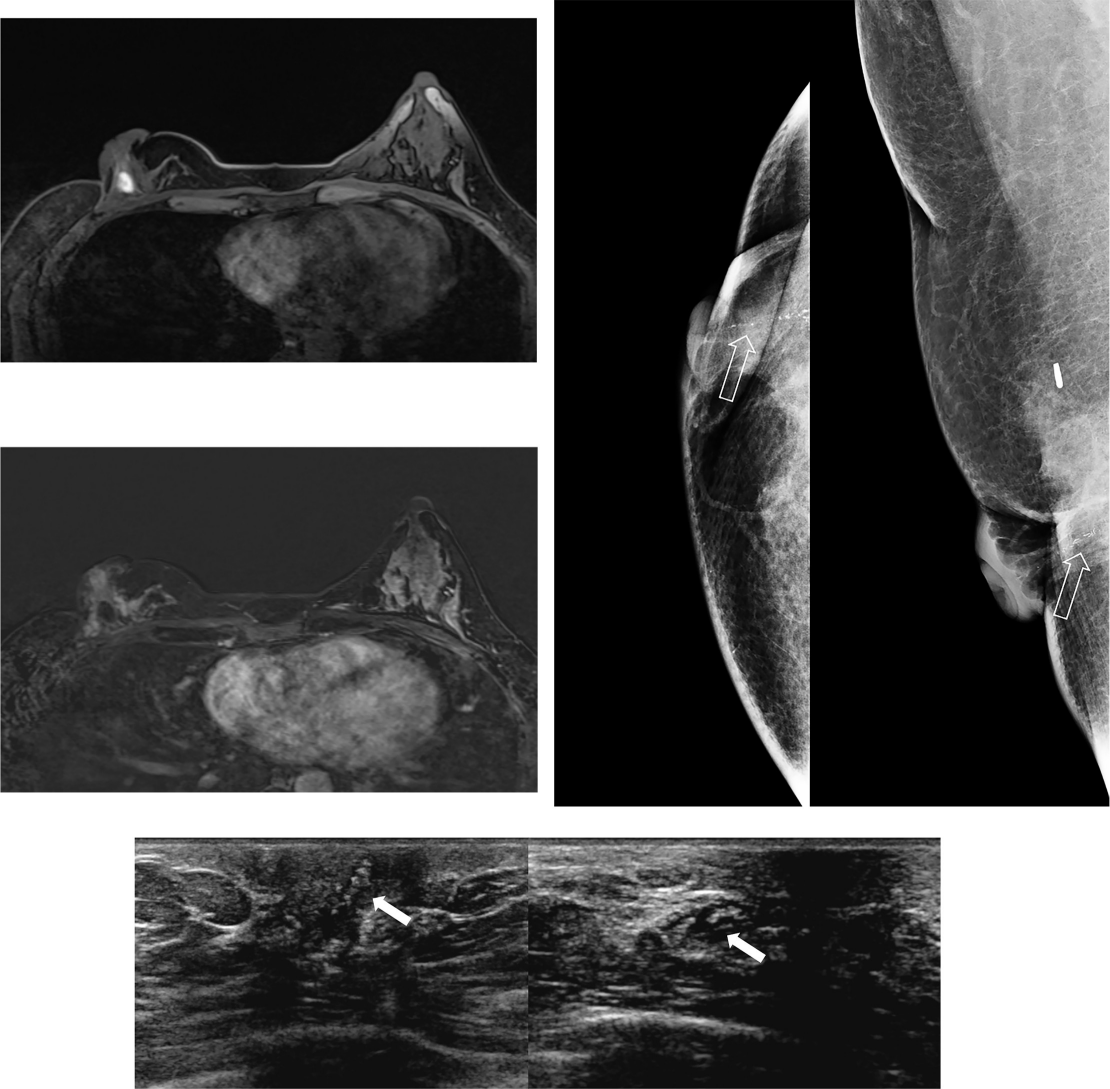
A 35-year-old female patient with a late recurrent breast cancer detected 30 months after BCT. (a), (b) MRI after recurrent ductal carcinoma in situ of right breast was confirmed through ultrasound-guided biopsy. Only hematoma is detected at right subareolar region on pre-contrast fat saturated T1WI (a), and no definite enhancement is noted on subtracted early dynamic phase of contrast enhanced fat saturated T1WI (b). (c) Mammography of the same patient for routine postoperative surveillance. Mammography showed linearly distributed fine pleomorphic microcalcifications (open arrow) at right subareolar region, adjacent to previous BCT site. (d) Ultrasound of the same patient for routine postoperative surveillance. Ultrasound showed intraductal echogenic foci (white arrow) at right subareolar region and nipple.
